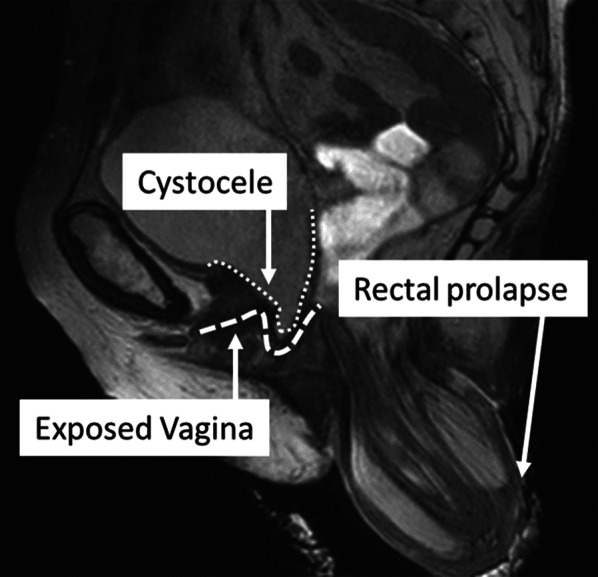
The magnetic resonance Image (MRI) revealed the cystocele and the exposed vagina with rectal prolapse
